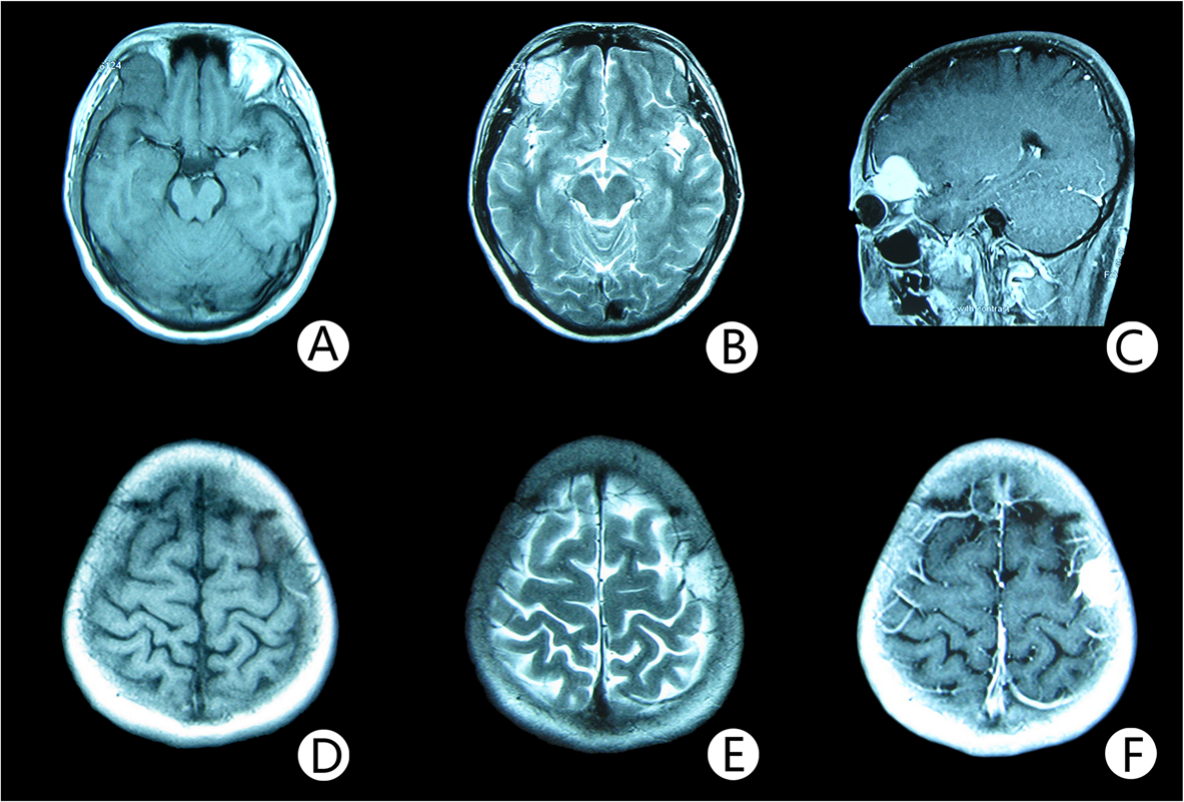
Magnetic resonance image features of the two lesions. Noncontrast axial T1-weighted MR image (A) reveals a well circumscribed mass, measuring 3 cm × 3 cm × 3 cm, in the upward wall of the right orbit with intracranial extension. The lesion is well defined, and the signal intensity is of mixed intensity with some central hyperintensity. T2-weighted MR image (B) shows a lesion of high signal intensity. There is no surrounding edema. After administration of gadopentetate dimeglumine (C), strong and homogeneous enhancement of the mass and a dural tail sign is observed. The lesion extended intracranially and appears to compress the underlying brain. The MR image of the mass in the frontal bone over the central sulcus (D; E; F) is similar to the one in the upward wall of the right orbit (A; B; C).
